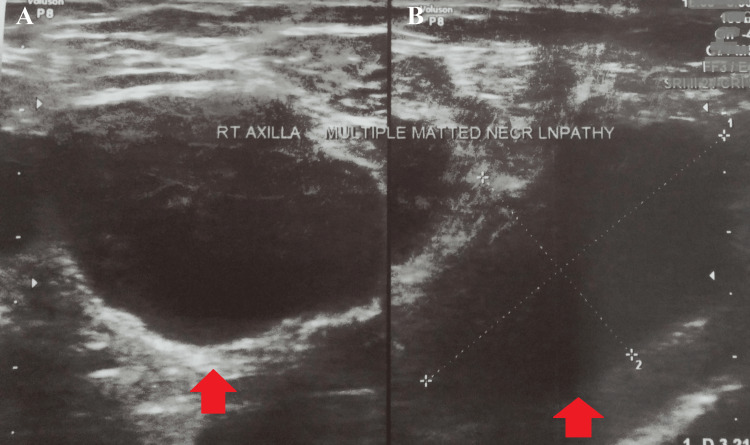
An ultrasound of the right axilla suggestive of a few enlarged axillary lymph nodes in the right axilla. A and B: axillary lymph nodes.
